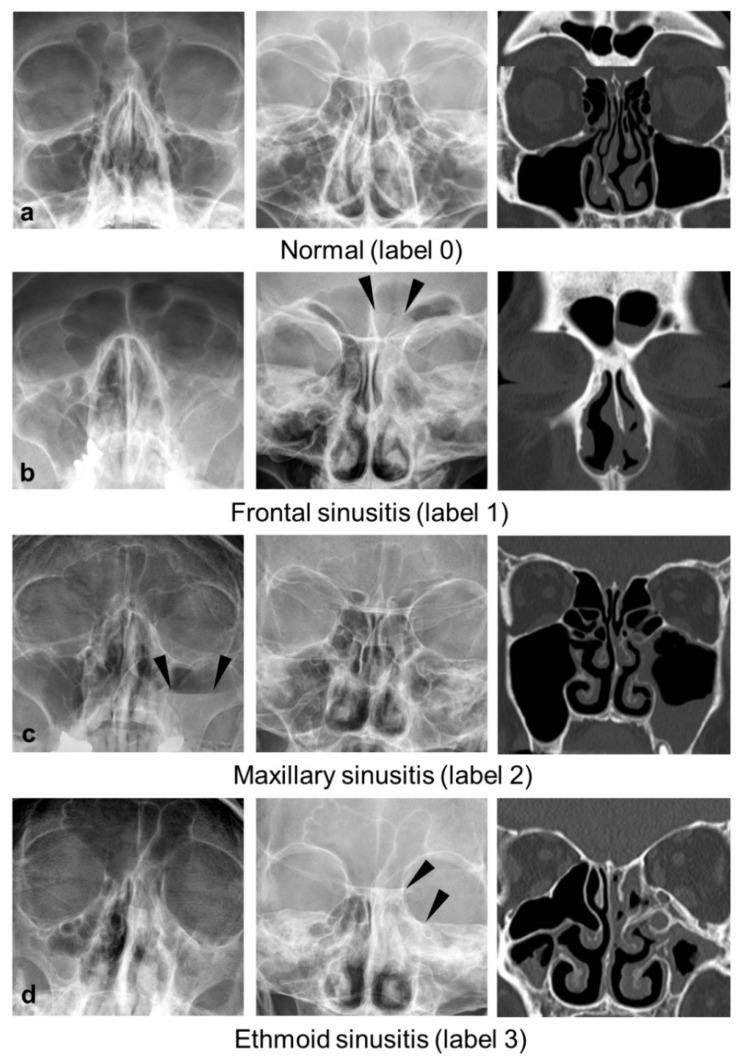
Representative cases with normal (label 0, a), frontal sinusitis (label 1, b), maxillary sinusitis (label 2, c), and ethmoid sinusitis (label 3, d) at each view (Waters’ view: left, Caldwell view: middle, corresponding coronal image of CT, right). For frontal (b) and ethmoid sinusitis (d), mucosal thickening (label 1) and total opacification (label 3) are not well visualized in Waters’ view, whereas Caldwell view provides the best projection for evaluation (arrowheads). In the case of maxillary sinusitis (c), Waters’ view provides a better view of the air-fluid level (label 2, arrowheads) than Caldwell view.
